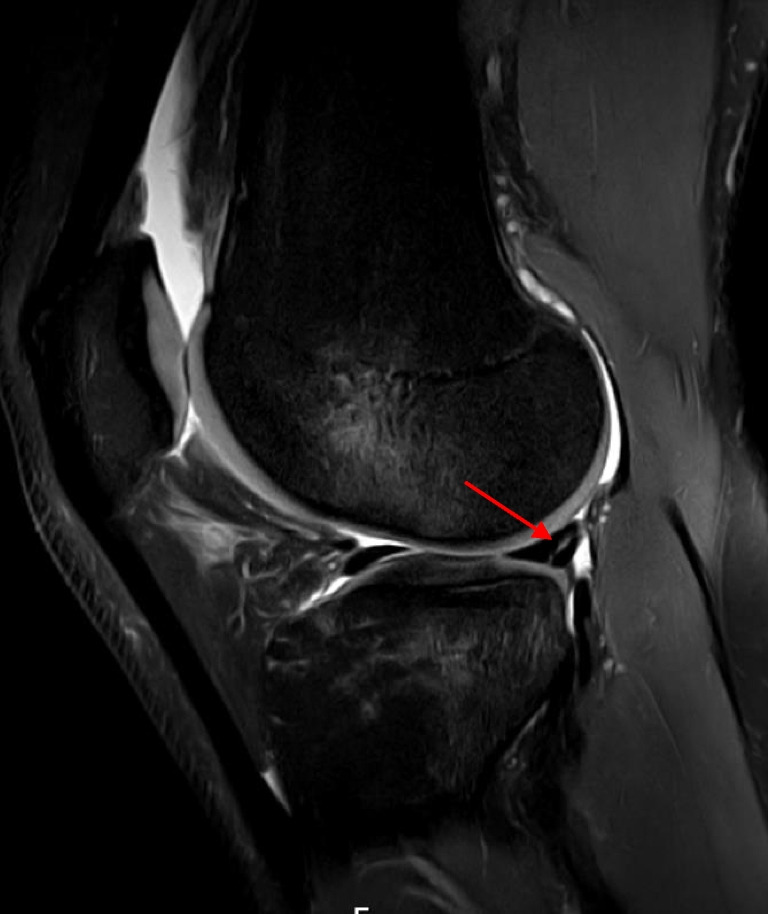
Radial-vertical lateral meniscus posterior horn tear (arrow) on sagittal proton density fat saturation magnetic resonance imaging sequence (right knee)
